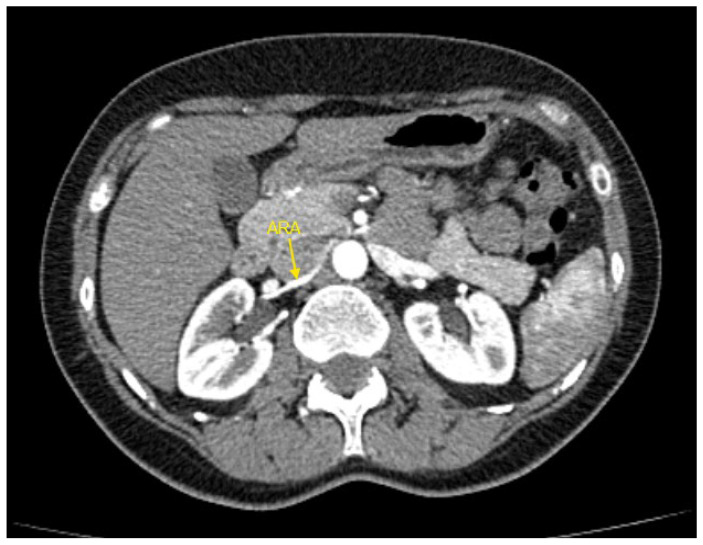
Contrast-enhanced CT imaging of a 49-year-old female hypertensive patient failing to achieve blood pressure control by 4 drugs from different classes demonstrated an accessory renal artery in the right kidney, indicated by the yellow arrow. ARA—accessory renal artery; CT—computed tomography.
